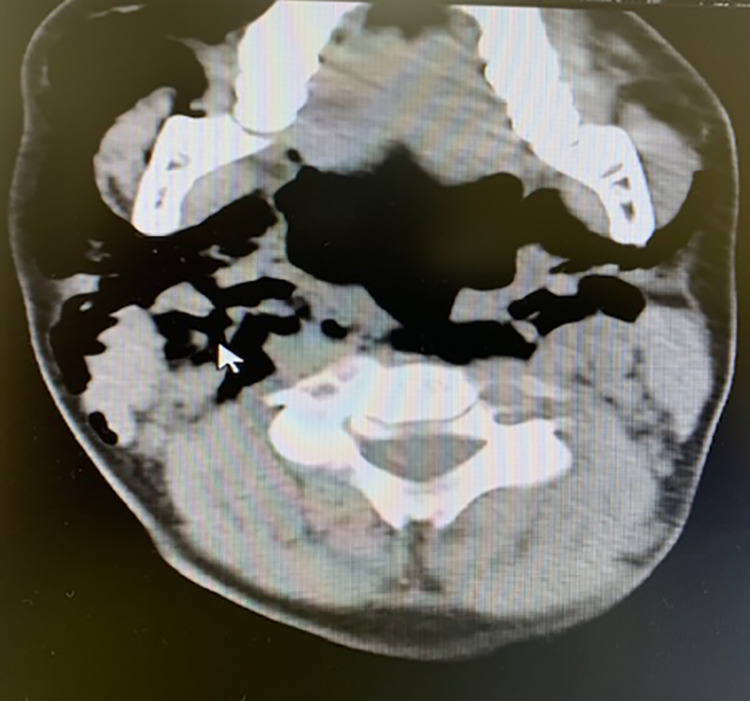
Non-contrast axial CT scan showing bilateral subcutaneous emphysema in the neck, more prominent in the right side, with mild pneumomediastinum.
